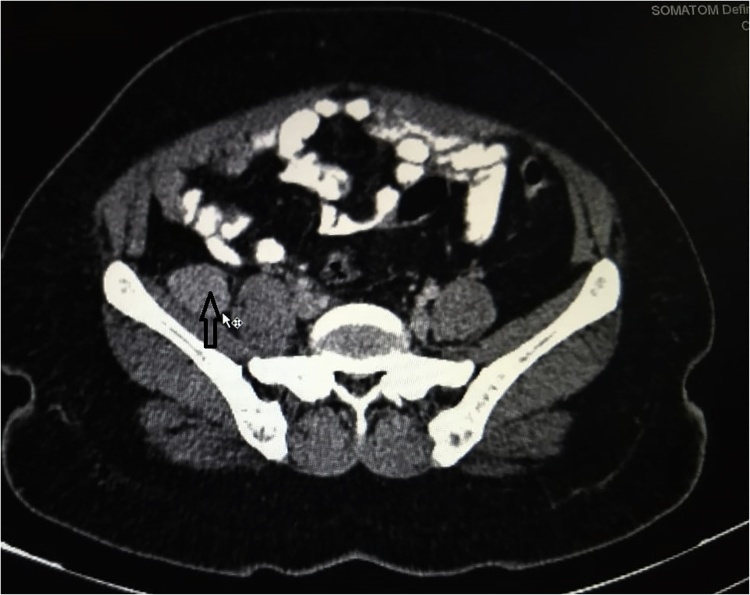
Retroperitoneal mass over right iliacus muscle. Contrast enhanced CT scan of abdomen showing homogeneous, vascular, well defined retroperitoneal solid mass overlying right iliacus muscle (arrow).
